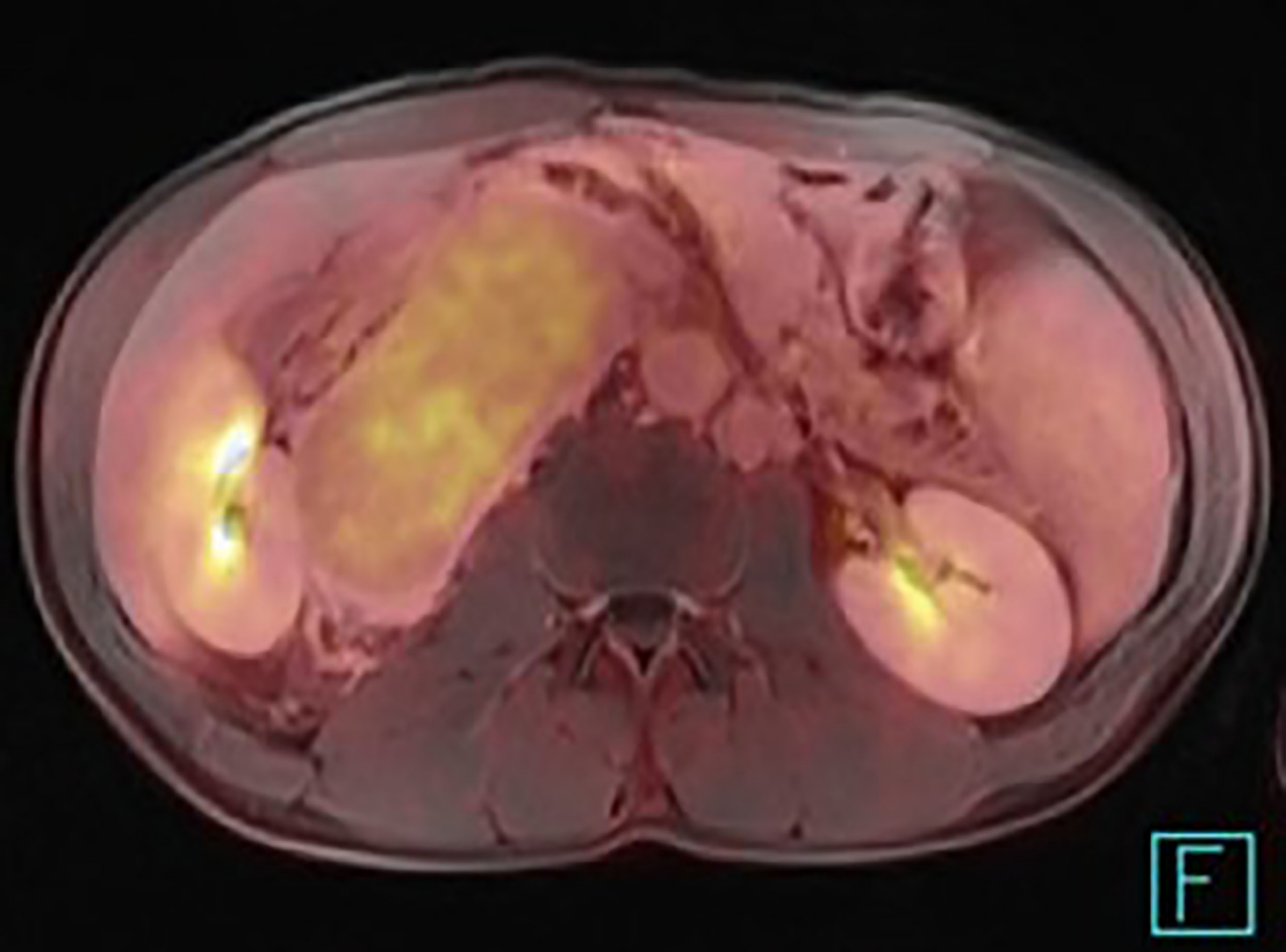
PET/MRI of the unicentric form of Castleman disease presenting a relatively low uptake of 18F-fluorodeoxyglucose.
